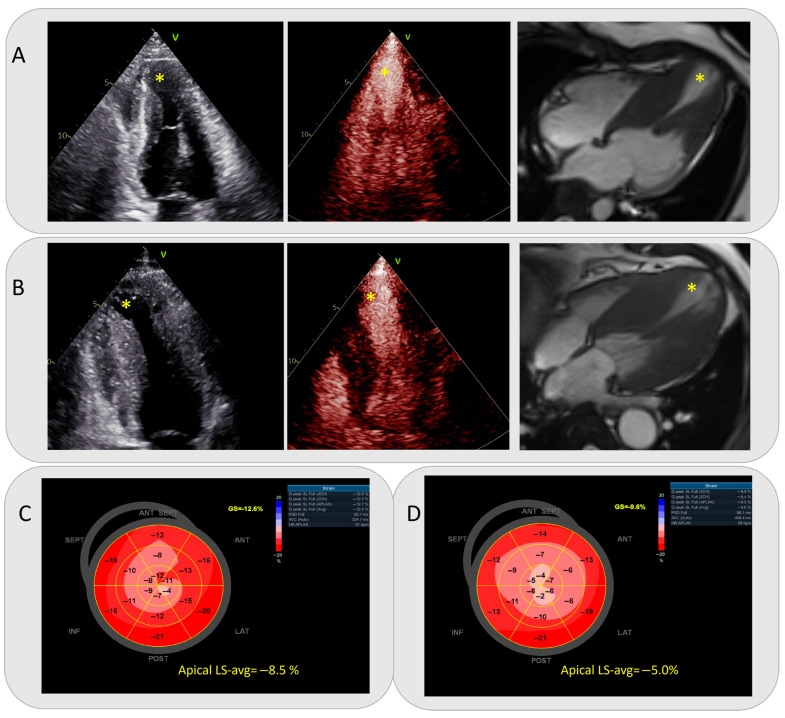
(A,C) A HCM patient with apical aneurysm did not experience any adverse cardiovascular events. A 20 mm apical aneurysm was revealed on two-dimensional transthoracic echocardiogram, contrast echocardiogram, and cardiac magnetic resonance, respectively. The patient’s 5-year SCD risk score was 3.0%, and apical LS-avg was −8.5% on a bull’s-eye map of speckle tracking imaging. The patient’s 5-year SCD risk score was 3.0%, and apical LS-avg was −8.5% on a bull’s- eye map of speckle tracking imaging. (B,D) Two-dimensional transthoracic echocardiogram, contrast echocardiogram, cardiac magnetic resonance image, and bull’s-eye map of speckle tracking imaging of an HCM patient with apical aneurysm who experienced adverse cardiovascular events. The patient had an apical aneurysm with a diameter of 23 mm, a 5-year SCD risk score of 3.4%, and an apical LS-avg value of −5.0%. The apical aneurysms on the images are marked with yellow asterisks. Abbreviations as in Table 1 and Table 2.
